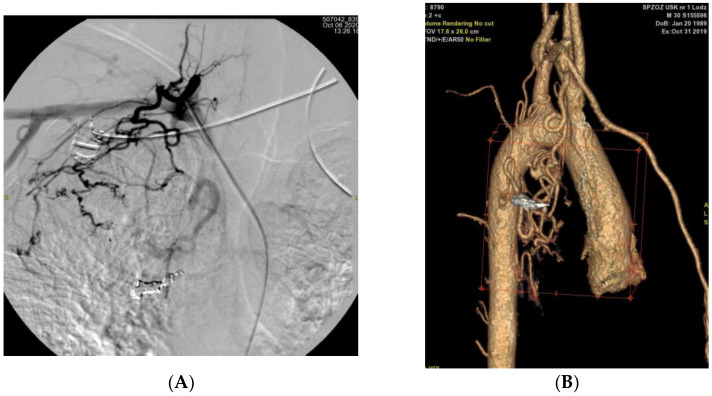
(A): Angiographic image obtained after bronchial artery embolisation, demonstrating the development of collateral vessels arising from the subclavian artery (B): Three-dimensional CT reconstruction of the aorta and its branches showing collateral formation originating from the subclavian arteries following bronchial artery embolisation.
